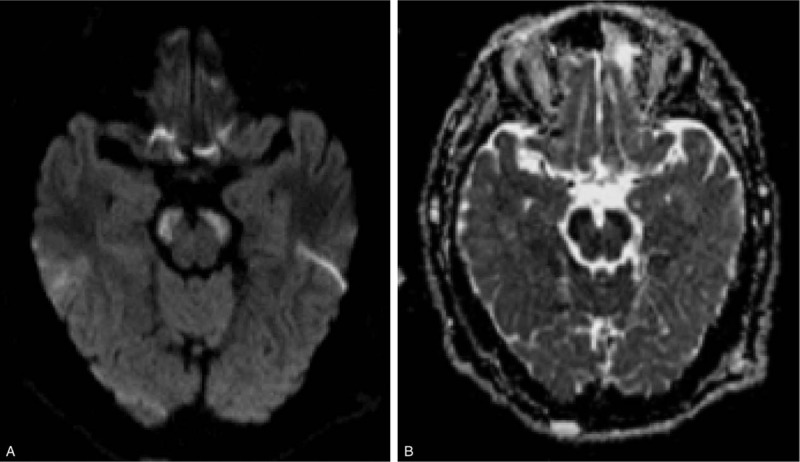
(A). Diffusion weighted imaging (DWI) performed upon admission showed a hyperintense bilateral peduncle cerebrum infarction; (B). With slight hypointensity in the apparent diffusion coefficient (ADC) map at the same position.
